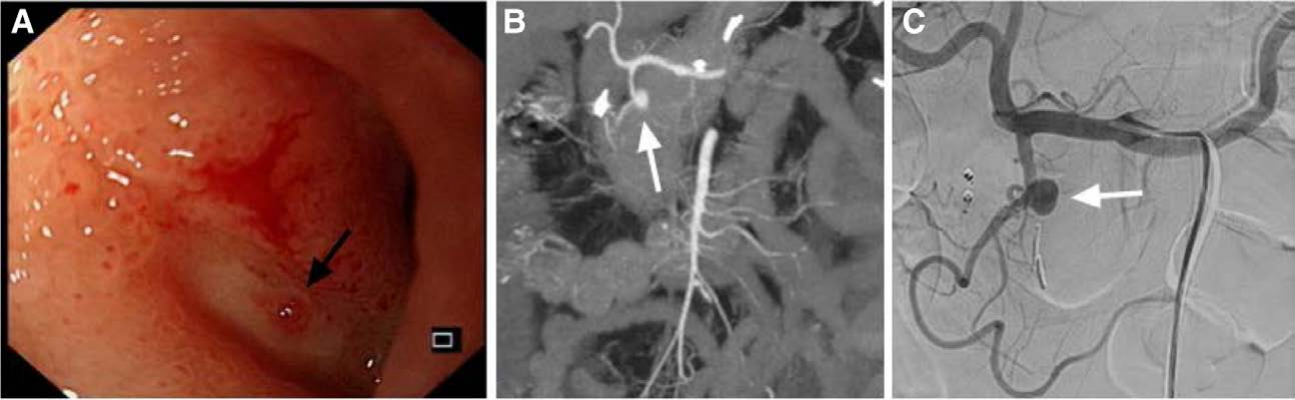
(A) Endoscopic view showed a white oval moss ulcer at the junction of D1 and D2 with vessel pulsating on its surface (black arrow); (B) Abdominal CT angiogram showed a significantly enhanced gastroduodenal pseudoaneurysm from the GDA (white arrow); (C) Gastroduodenal angiography confirmed the gastroduodenal pseudoaneurysm (white arrow). CT = computerized tomography, D1 = the first part of the duodenum, D2 = the second part of the duodenum, GDA = gastroduodenal artery.
